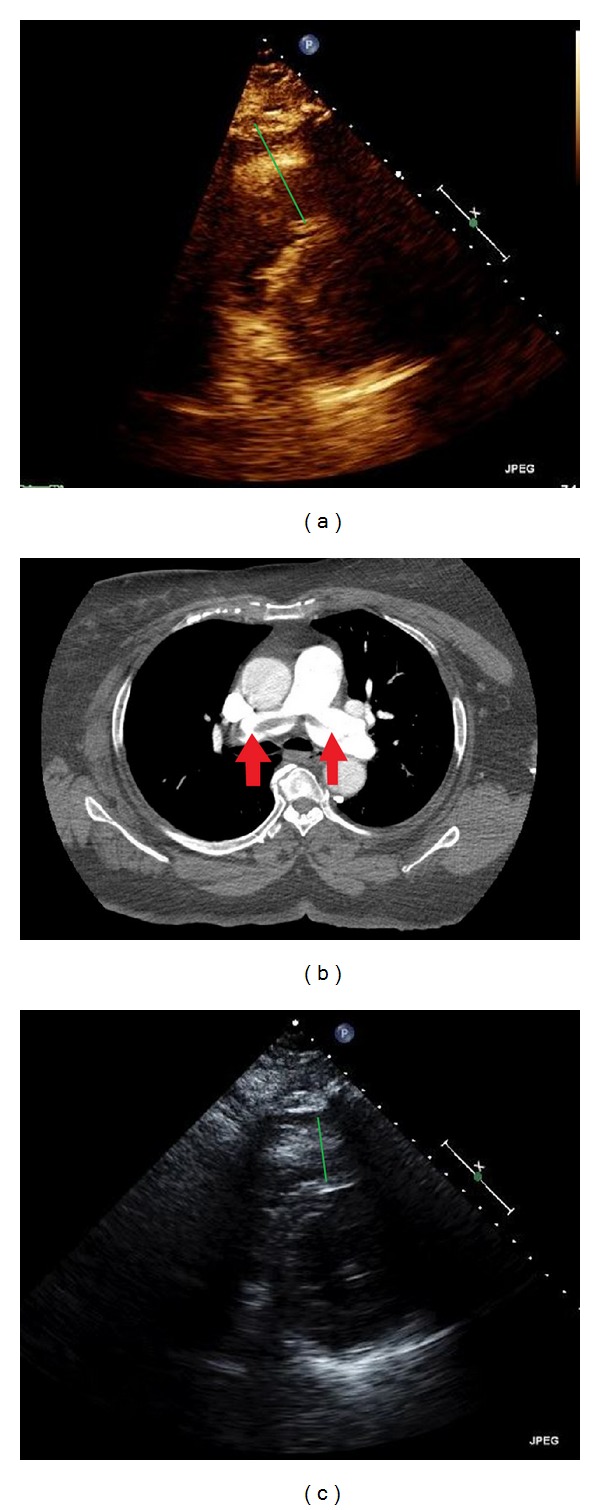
(a) The echocardiogram shows dilated right ventricle (green line). (b) The CT-scan shows an evidence of saddle emboli (red arrows). (c) There was a marked improvement in right ventricle size after the treatment (green line).
